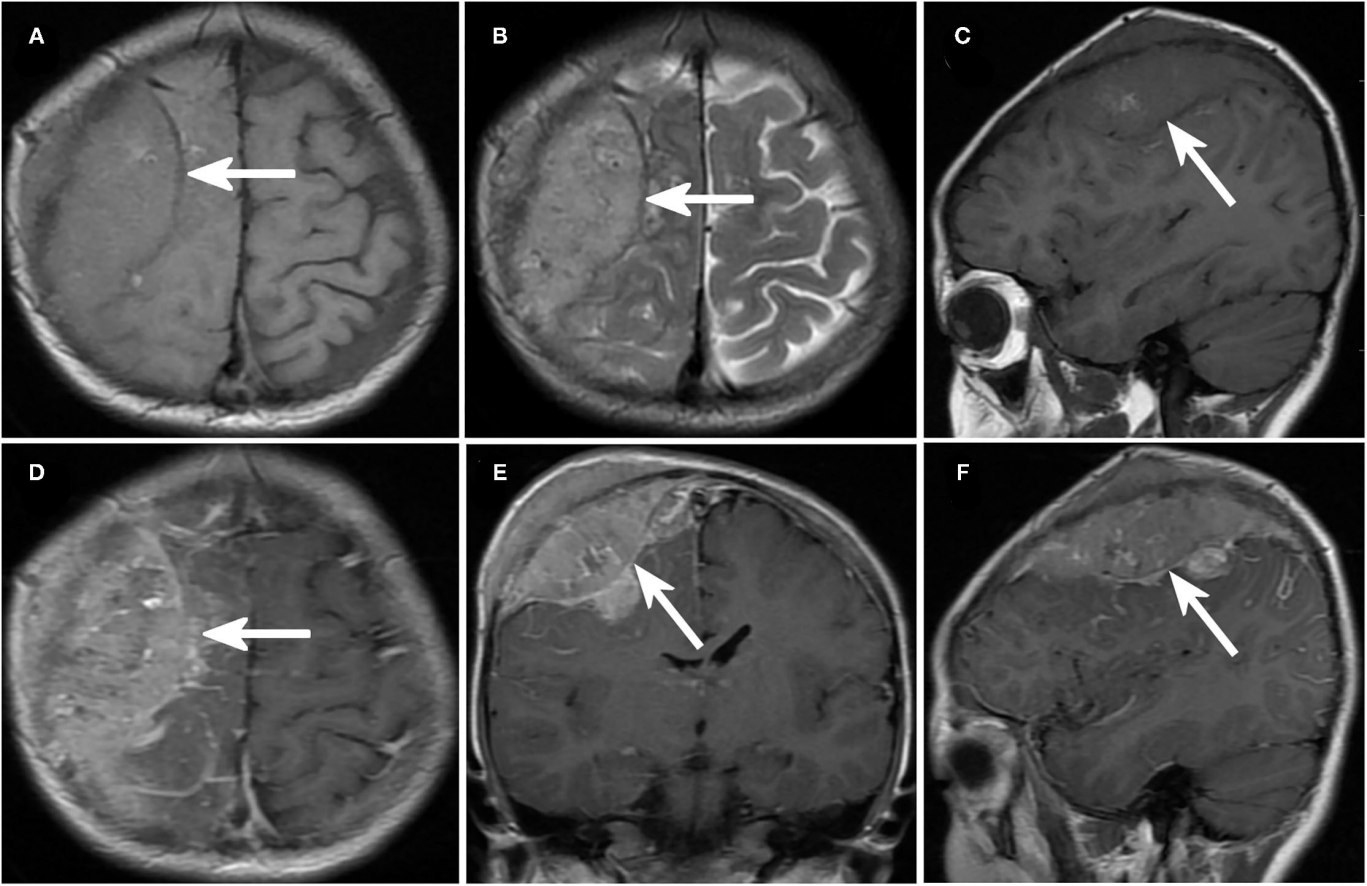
Imaging of neuroblastoma with intracranial metastases. (A–C) Axial (A,B) and sagittal (C) magnetic resonance imaging (MRI) in case No. 11 demonstrated a recurrent mass lesion in the right occipital lobe. (D–F) Cranial enhanced MRI of case No. 11, axial (C), coronal (D), and sagittal (F) images.
